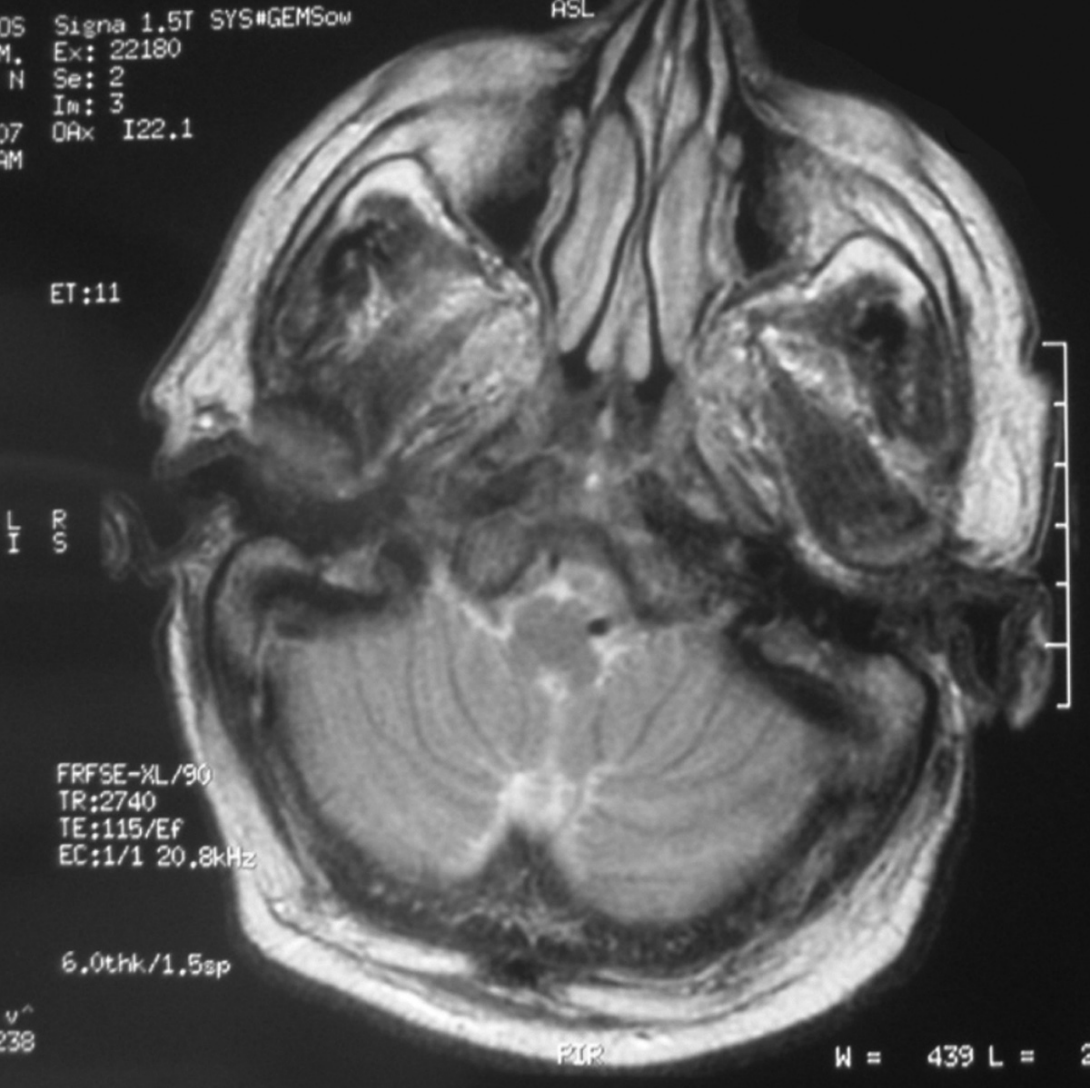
Magnetic resonance imaging scan without apparent alterations.
